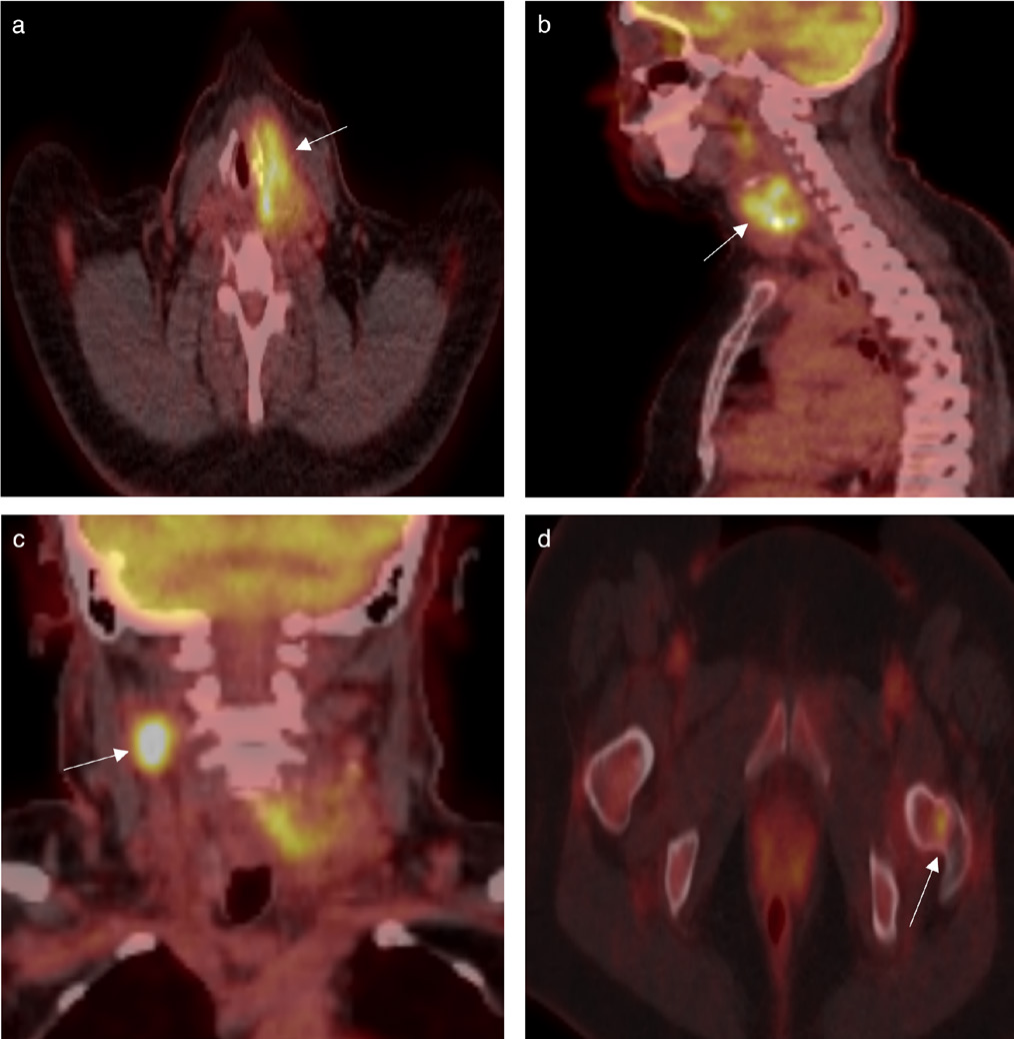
FDG PET scan CT axial (a) and sagittal (b) images confirm high grade metabolic activity extending along the left posterolateral hypopharyngeal wall indicating diffuse infiltration by the primary lesion. (c) FDG PET CT coronal image confirms high grade metabolic activity in a right level two lymph node indicative of nodal metastasis. (d) FDG PET CT axial image demonstrates focal increased metabolic activity in the left femoral neck raising suspicion for skeletal metastasis. FDG, fludeoxyglucose; PET, positron emission tomography.
